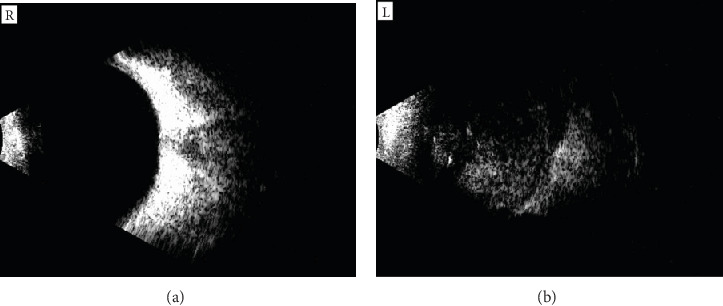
Ultrasound B-scan findings. (a) Normal appearance of the right eye. (b) Left eye demonstrating extensive vitreous infiltration by a solid mass, characterized by hyperechoic lesions and isolated calcified areas.
